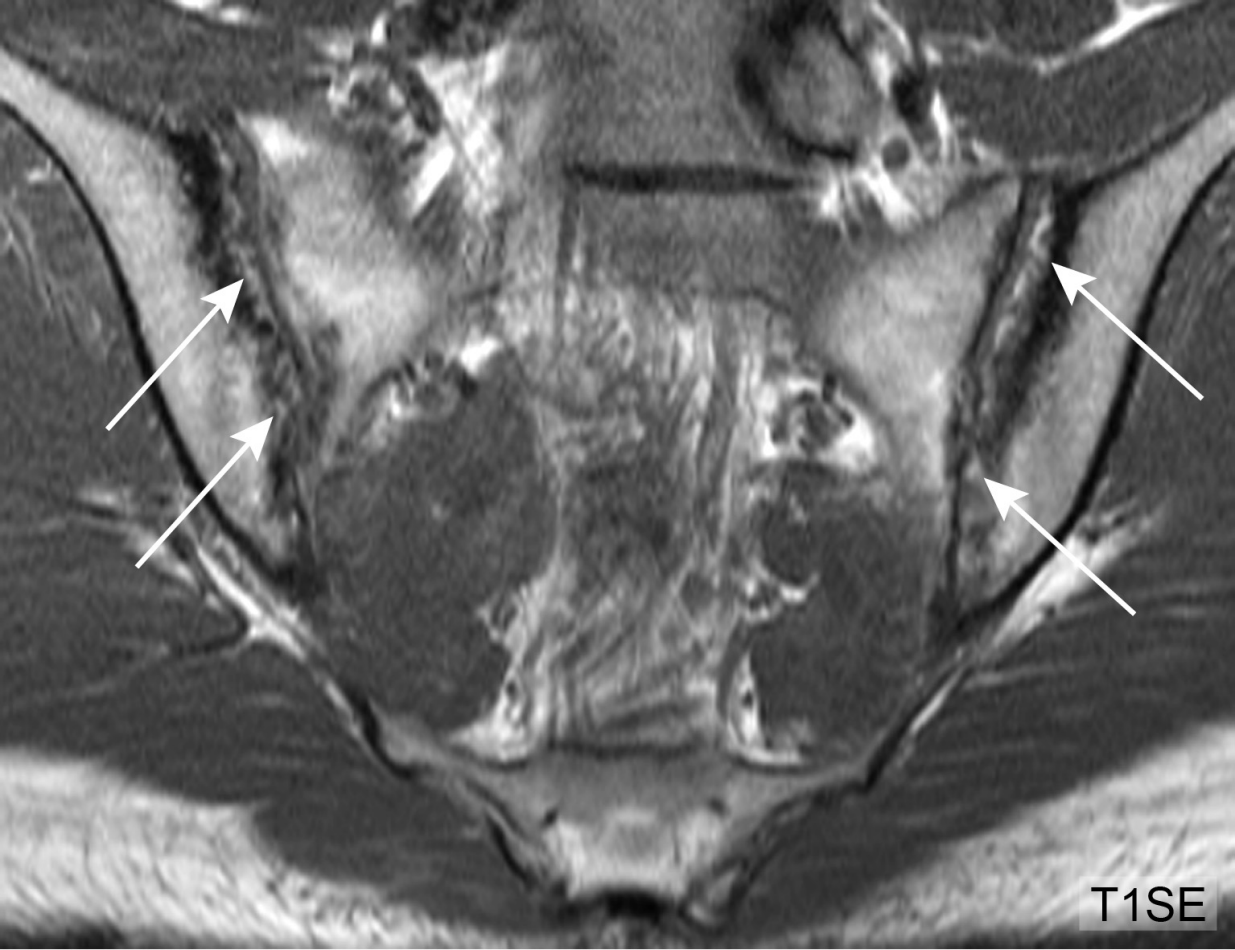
Backfill, baseline MRI (T1SE sequence). HLA B27 positive, 25-year-old male AS patient. The T1SE sequence of the SIJ displays extended erosion of the iliac joint portion on both sides. The excavated iliac bone is replaced by tissue showing MR signal intensity similar to or higher than normal bone marrow (arrows). HLA-B27, human leucocyte antigen B27; MRI, magnetic resonance imaging; T1SE, T1-weighted spin echo sequence.
